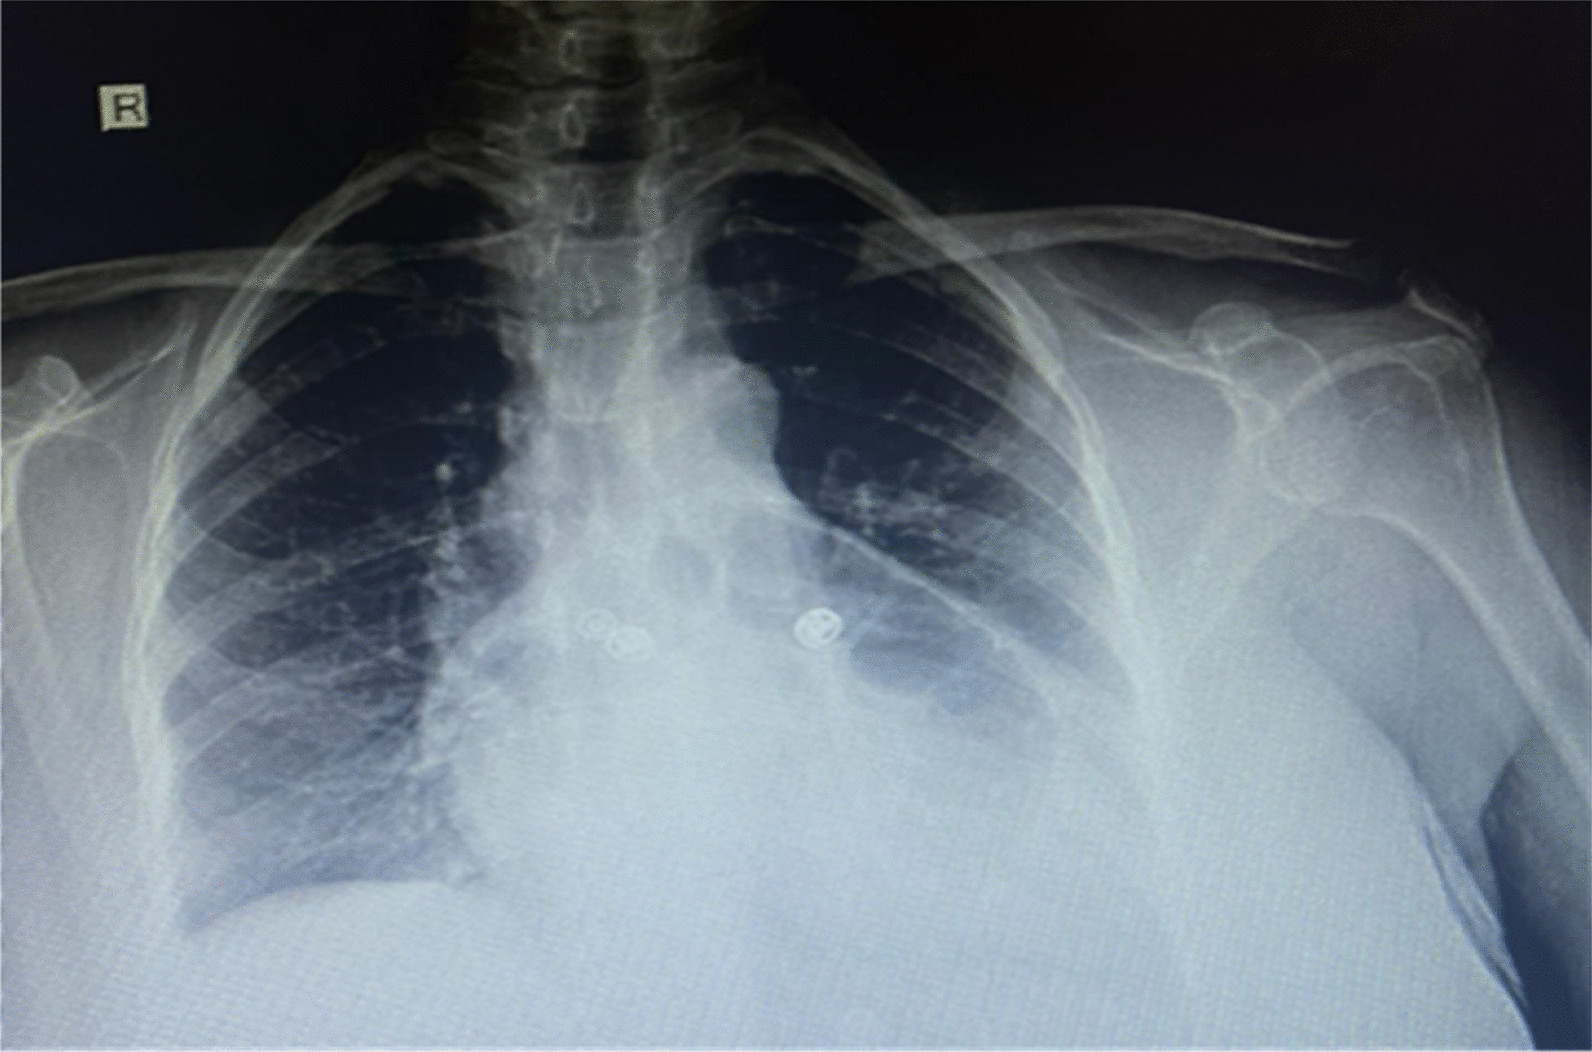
Preoperative chest radiography with a wide mediastinum and an air-containing viscus indicating intrathoracic intestine loops
